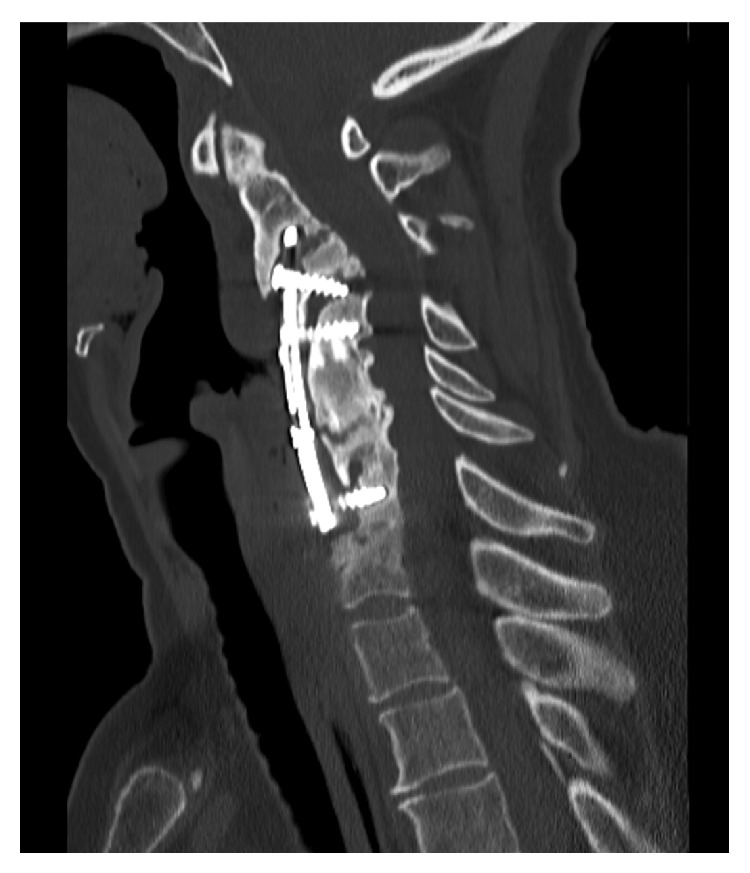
Sagittal CT view shows evidence of pseudarthrosis, lucency around screws at C3 and C7, and anterolisthesis of C2 in relation to C3, with the C2 vertebra overriding the superior aspect of the metallic plate.
